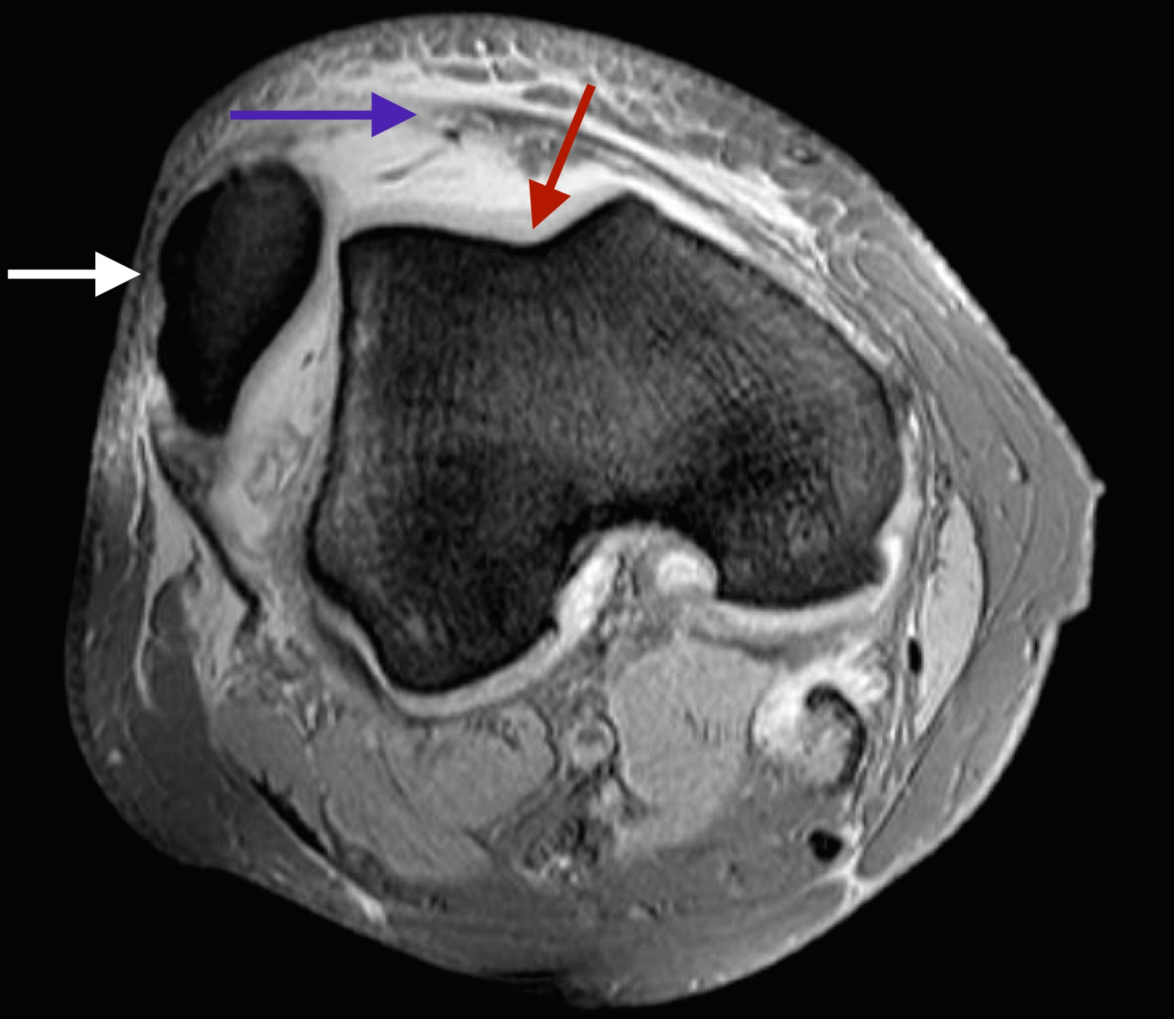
Axial magnetic resonance image of right knee showing lateral patellar dislocation (white arrow), bony avulsion of medial patellofemoral ligament (purple arrow) and a raised sulcus angle (red arrow).
